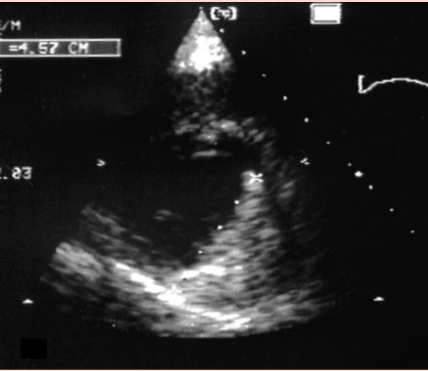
Parasternal short axis recording of the left ventricular cavity. The tumor– semilunar shape, extended from one o'clock to seven o'clock. The same homogenous, uniform echostructure of the mass
